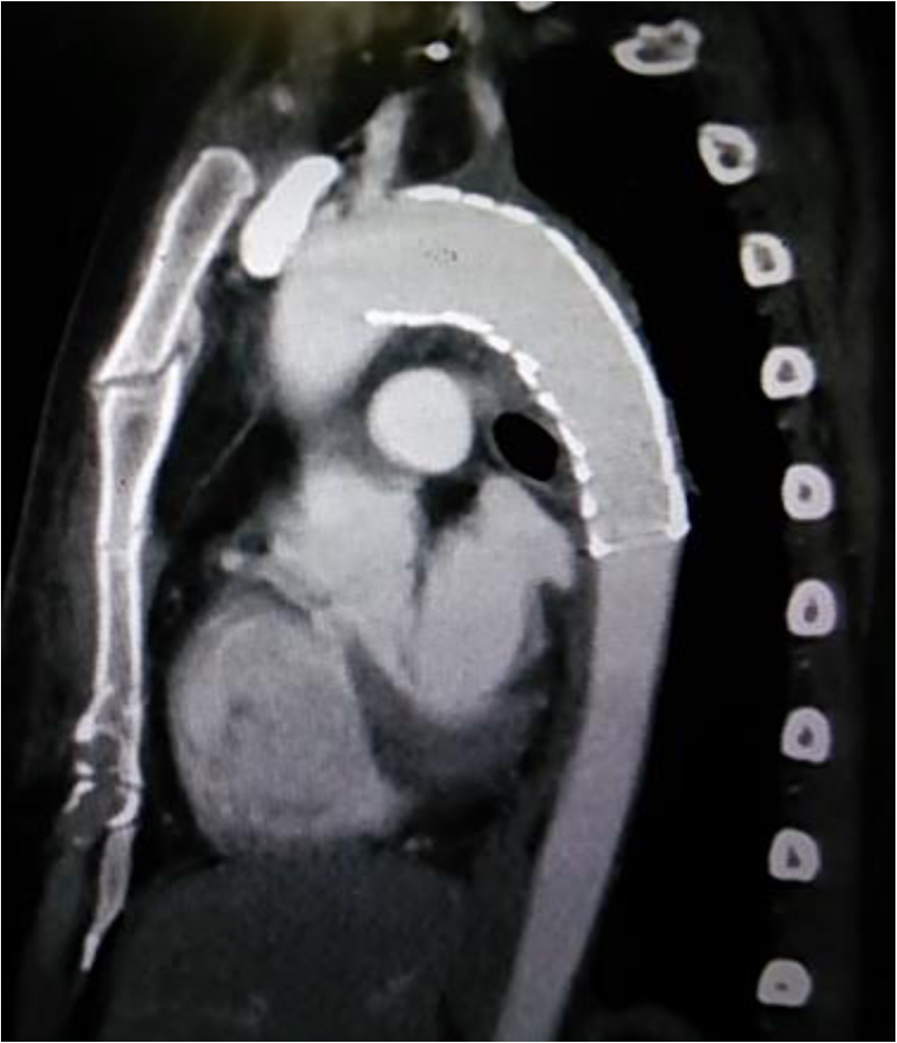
CT image after stent grafting showed no evidence of endoleak, and the stent was in a good position
